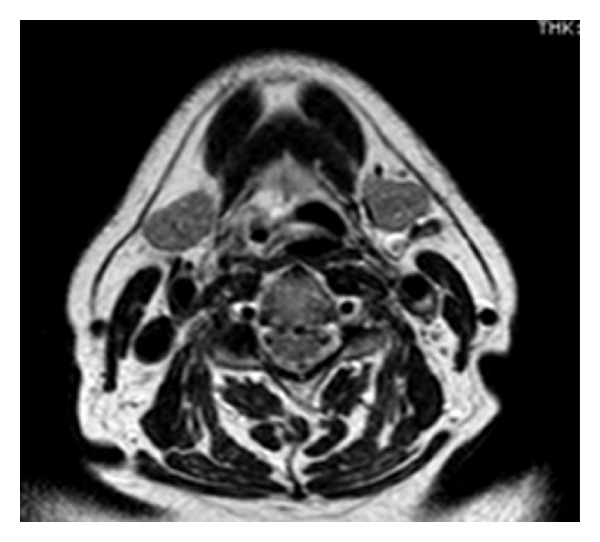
MR image shows an irregular area of tissue thickening with moderate contrast enhancement on the right pharyngo-epiglottic ligament and omolateral pyriform sinus.
